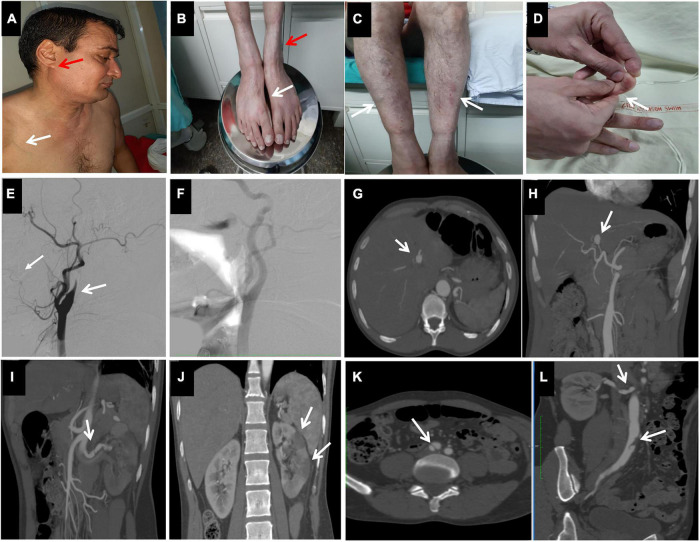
Observed clinical features of the patient, (A): Thin Nose, lobe less ear (red arrow), thin skin with visible chest veins (white arrow), (B): Acrogeria (red arrow) with club foot (white arrow), (C): Easy bruising and thin skin, varicose veins, (D): Hypermobility of small joints (shown in distal phalange of index finger). Digital subtraction angiography images of bilateral common carotid arteries showing dissection of left internal carotid artery (E,F). Right internal carotid artery was normal. Axial (G) and coronal (H) maximum intensity projection (MIP) CT images showing aneurysmal dilatation of left hepatic artery. Coronal MIP images (I,J) of contrast enhanced CT show dissection involving left renal artery with multiple infarcts of renal parenchyma. Axial (K) and coronal (L) MIP CT images show dissection involving right renal artery and right common iliac artery.
